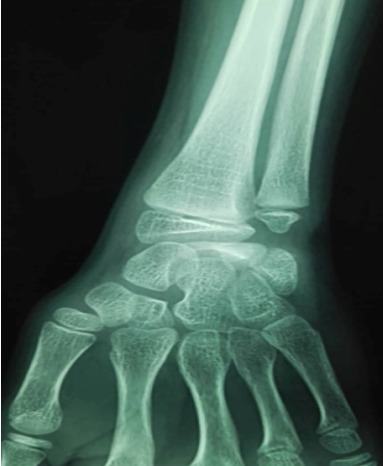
Face radiograph shows collapse of the lunate
